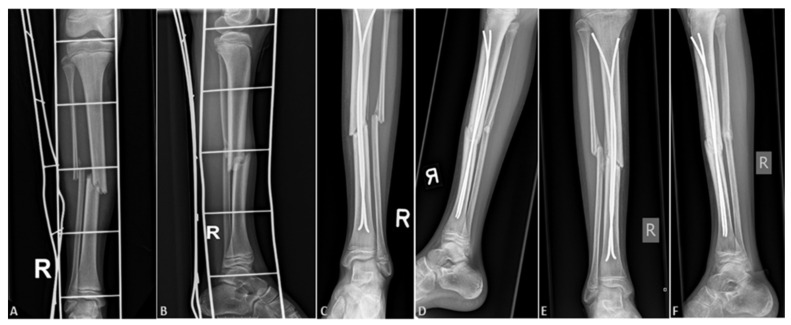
Displaced tibial shaft fracture in 11-year-old female patient: (A) preoperative AP radiograph; (B) preoperative LL radiograph; (C) AP radiograph one month after surgery; (D) LL radiograph one month after surgery; (E) AP radiograph three months after surgery; (F) LL radiograph three months after surgery.
